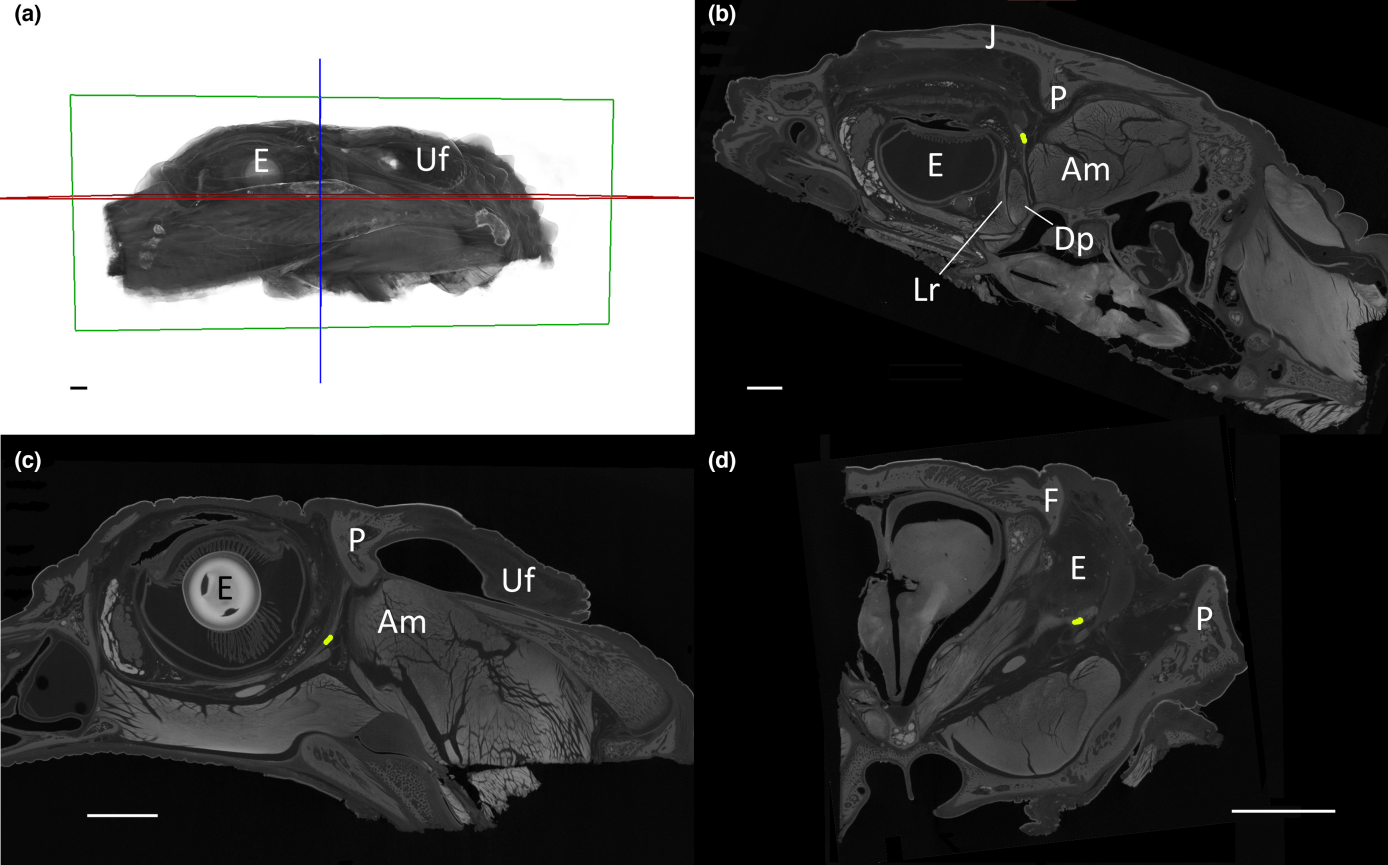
Anatomical position of the orbitalauricular chord. (a) 3‐D reconstruction of the sample used for the micro‐CT imaging; the planes for the other three images are color‐coded. Images from the frontal (b), sagittal (c), and transverse (d) planes; the point of intersection of the three planes falls on the orbitalauricular chord and is highlighted in yellow. Scale bars are all 5 mm. Am, adductor mandibulae externus superficialis; Dp, Depressor palpebra; E, eye; F, frontal; J, jugal; Lr, lateral rectus; P, postorbital; Uf, upper earflap.
